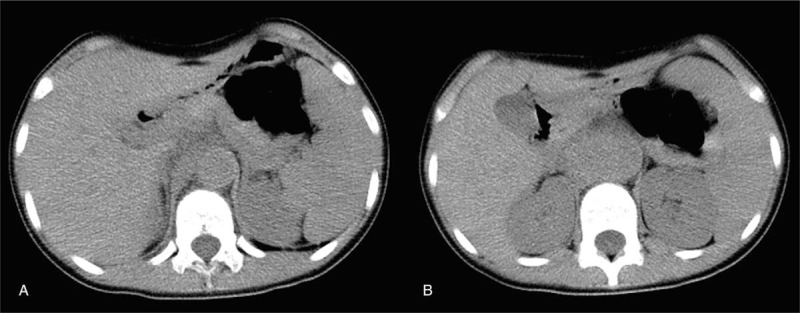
Abdominal CT scan: Enlargement of the upper abdominal aorta, approximately 25.4 mm in diameter (A). The abdominal aorta could not be clarified at the level of the kidney and appeared as mass opacities in the size of 49.6 × 32.3 mm (B).
